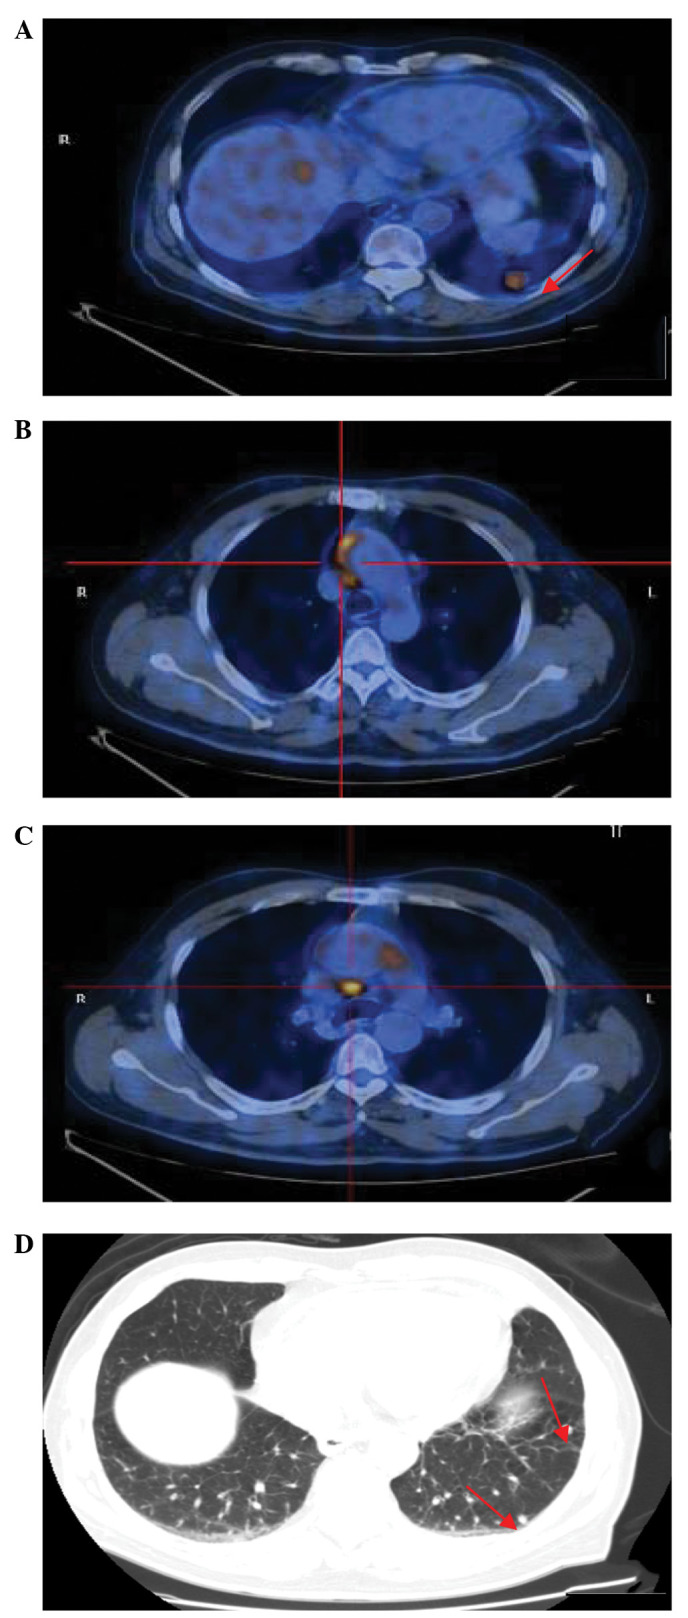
Nodules revealed by ^18^F-fluorodeoxyglucose positron emission tomography/computed tomography (^18^F-FDG-PET/CT) (August 3, 2011). (A) The largest nodule is visible at the basal segment of the left lower lobe [maximum standardized uptake value (SUV_max_), 5.8]. (B) Nodules observed in the ascending aorta/aortic arch wall (SUV_max_, 6.4). (C) Nodule observed in the pericardial wall (SUV_max_, 8.3). (D) Nodules observed in the left lower lobe (^18^F-FDG uptake was normal).
